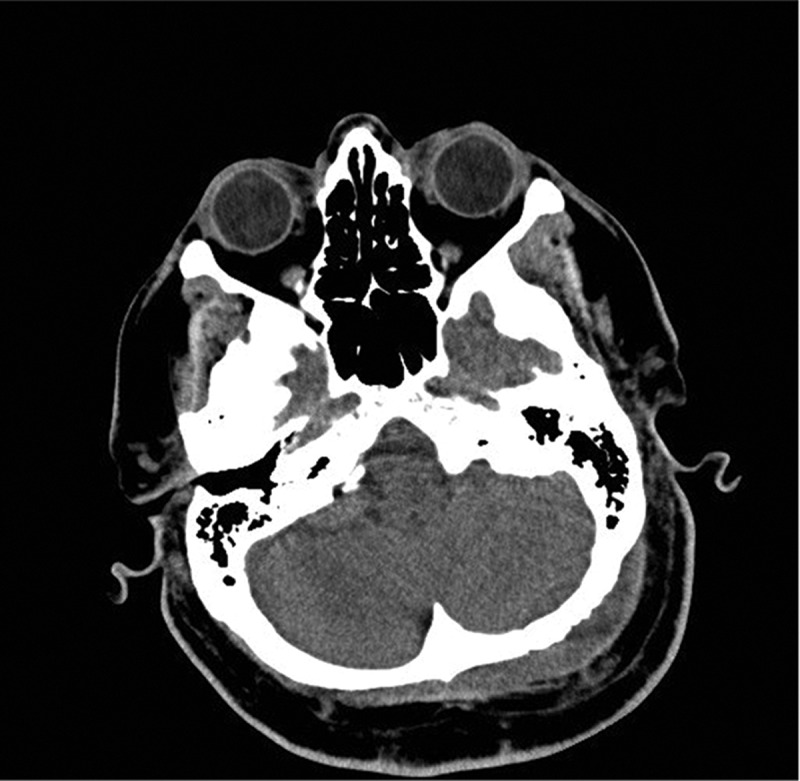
CT scan showing soft tissue swelling suggestive of epidural abscess of left posterior fossa.
